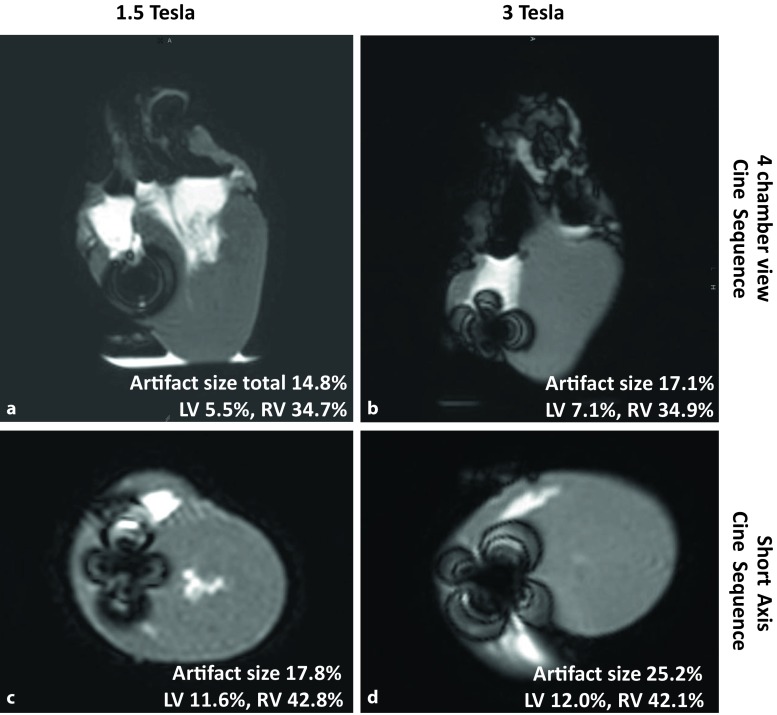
Magnetic resonance imaging in “cine-like” sequences (SSFP-sequence) showing a “shamrock-shaped” artifact masking a small focal area without compromising the surrounding myocardium. The artifact compared to images obtained at 1.5 T (a 4-chamber view, c short axis view), analysis at 3.0 T showed evidence of a larger visual artifact (b 4‑chamber view, d short axis view)
